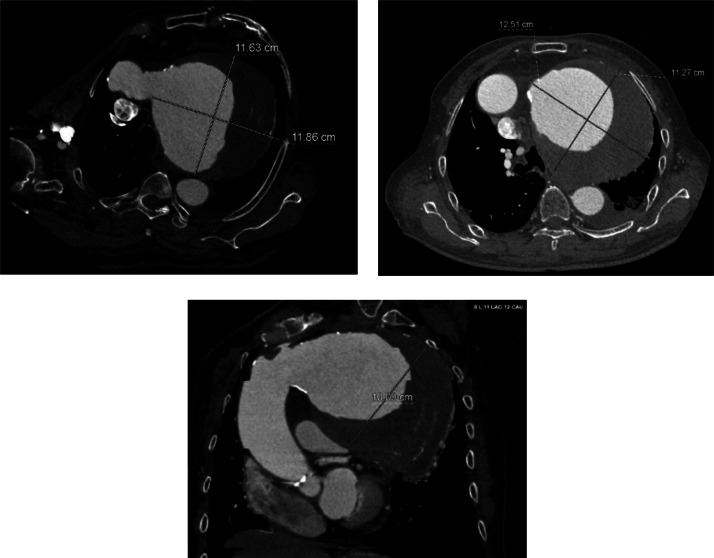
Cardiac computed tomography scan with contrast showing giant ascending aortic aneurysm.
